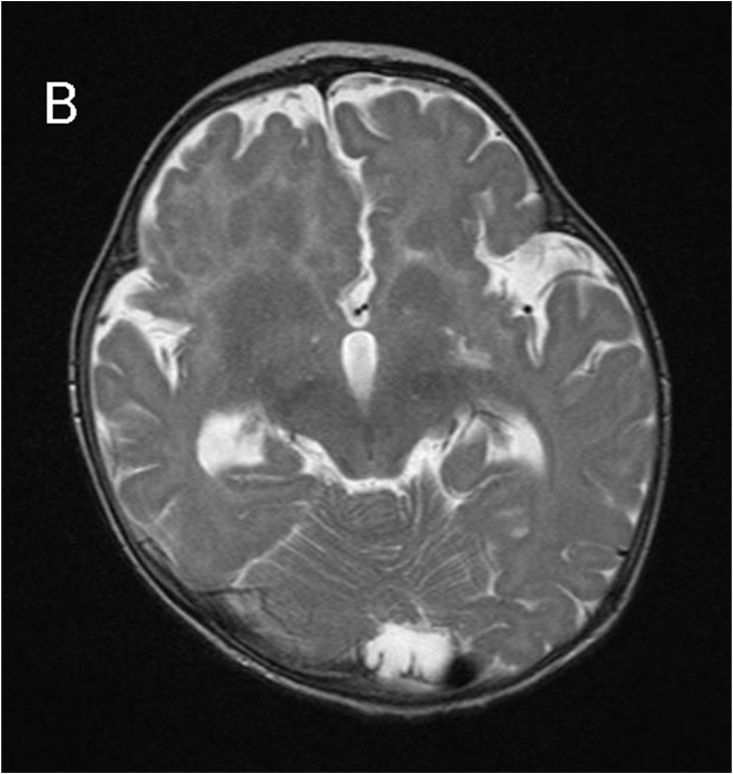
transverse T2-weighted image showing underdevelopment of the left operculum.
